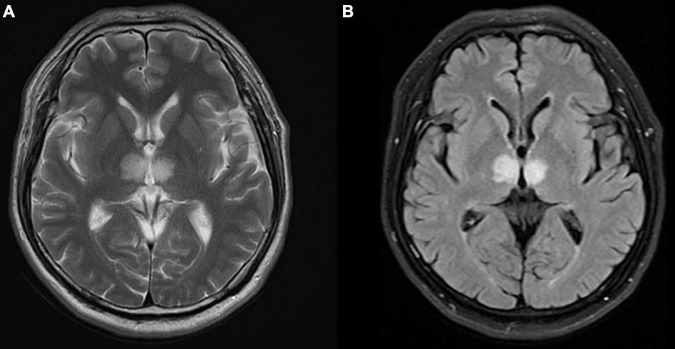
Axial magnetic resonance imaging (MRI) presenting bilateral high-signal intensity on the paramedian thalamus in (A) T2-weighted sequence, and (B) FLAIR sequence.
